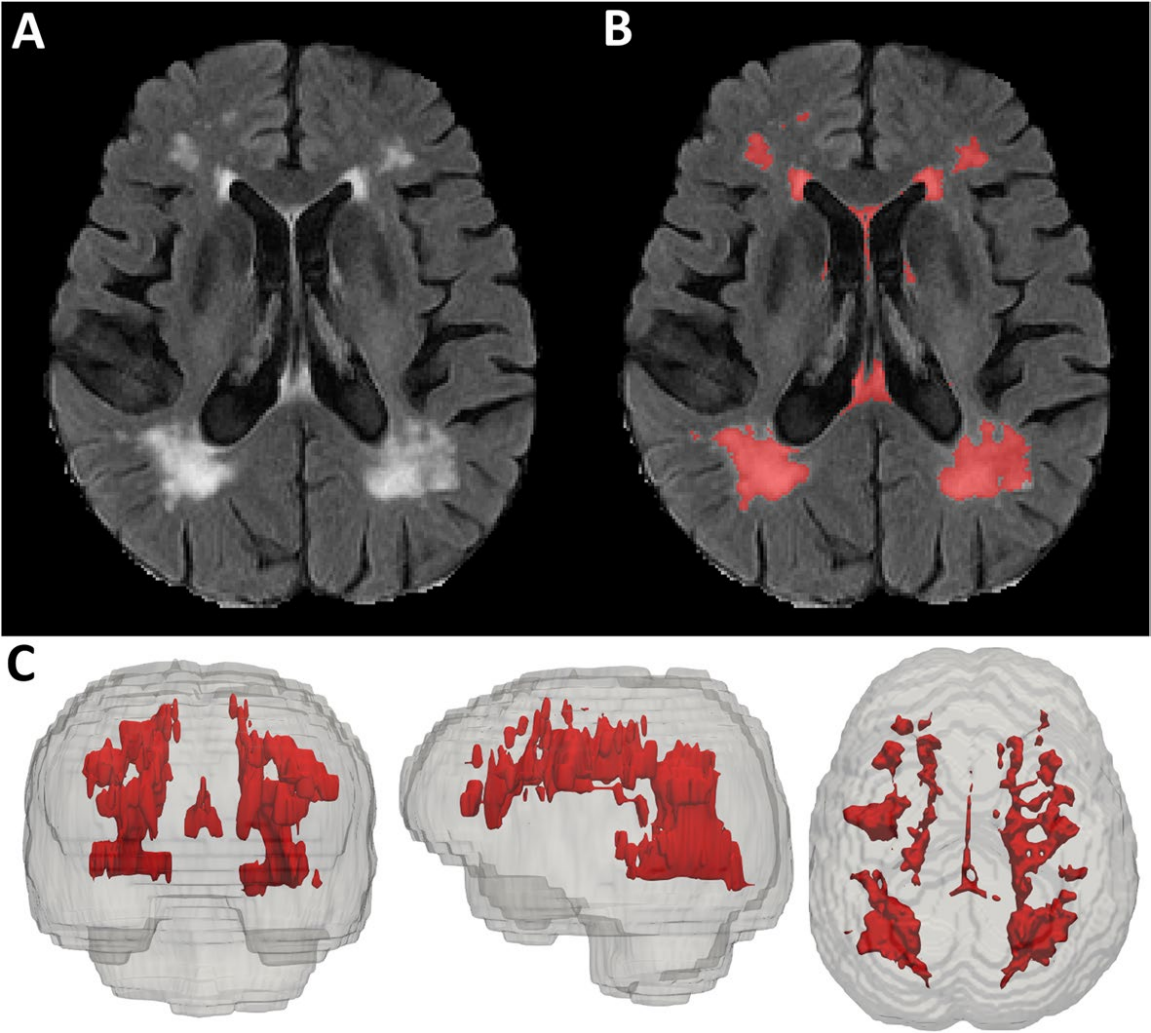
Example of a raw (A) and labeled for WMH (B) T2-weighted FLAIR image on a single axial slice from an A4 study participant. C 3D rendering of total WMH in same participant in coronal, sagittal, and axial view, respectively
